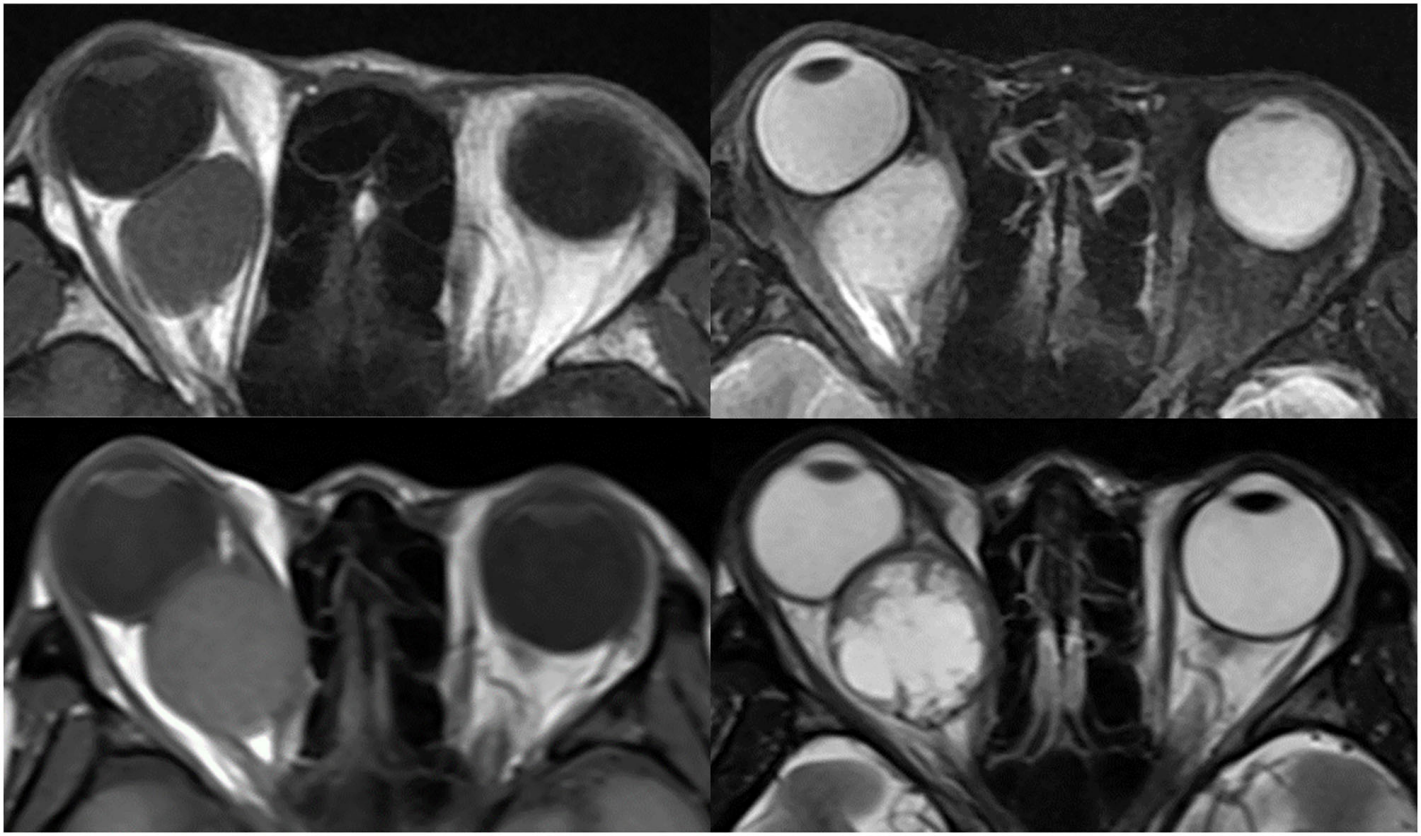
Example images of OCH and OSC. Top left is a T1-weighted image of a patient with OCH, top right is a T2-weighted image of the same patient. Bottom left is a T1-weighted image of a patient with OSC, bottom right is a T2-weighted image of the same patient.
